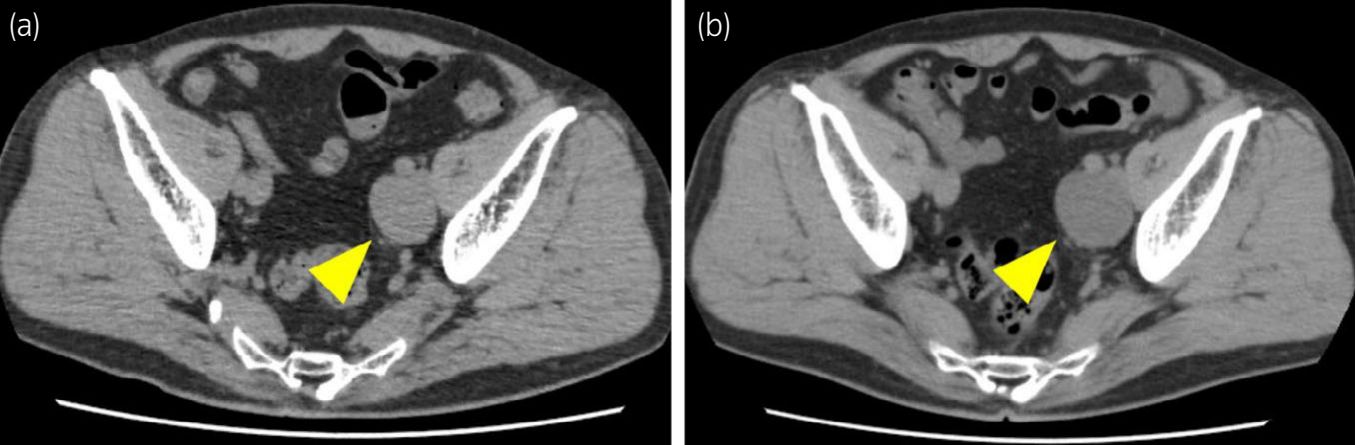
(a) CT scan prior to the initiation of degarelix acetate. Clinical recurrence of PCa. (b) CT scan after the initiation of degarelix acetate, which revealed the enlargement of metastasis.
